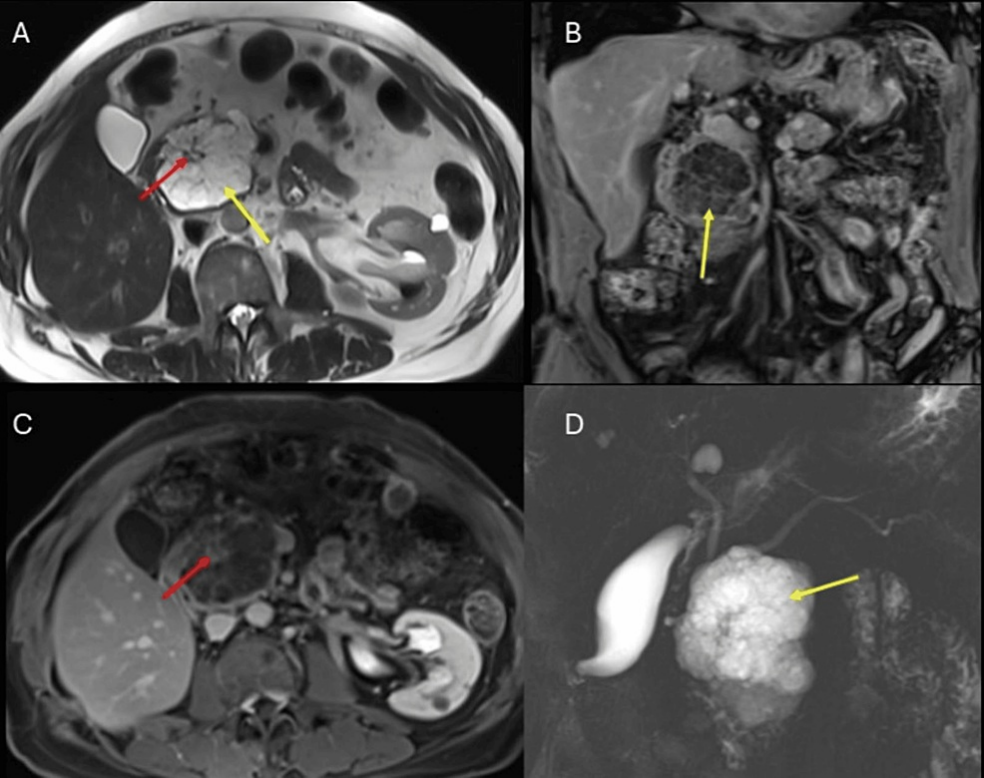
Serous cystic tumor of the pancreas. Contrast-enhanced magnetic resonance imaging of the abdomen. A: T2-weighted sequence in the axial section; B: T1-weighted sequence after gadolinium administration in the coronal section; C: T1-weighted sequence after gadolinium administration in the axial section. D: Cholangioresonance sequence. A lesion is observed in the head of the pancreas, made up of multiple confluent cysts (yellow arrows) and thin septa inside that converge in a central scar and present enhancement with the contrast medium (red arrows). Findings compatible with a serous cystic tumor of the pancreas.
